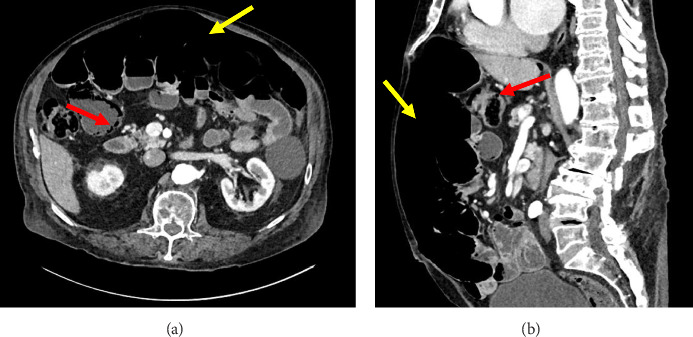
Initial ED presentation CT abdomen and pelvis with contrast: (A) Axial view demonstrating large anterior pneumoperitoneum (yellow arrow) with pneumatosis intestinalis (red arrow) present in a dependent portion of the small intestine. (B) Sagittal view demonstrating large anterior pneumoperitoneum (yellow arrow) spanning from the distal-most aspect of the abdomen to the diaphragm. Small pneumatosis intestinalis (red arrow) is also present in a dependent portion of the small intestine.
